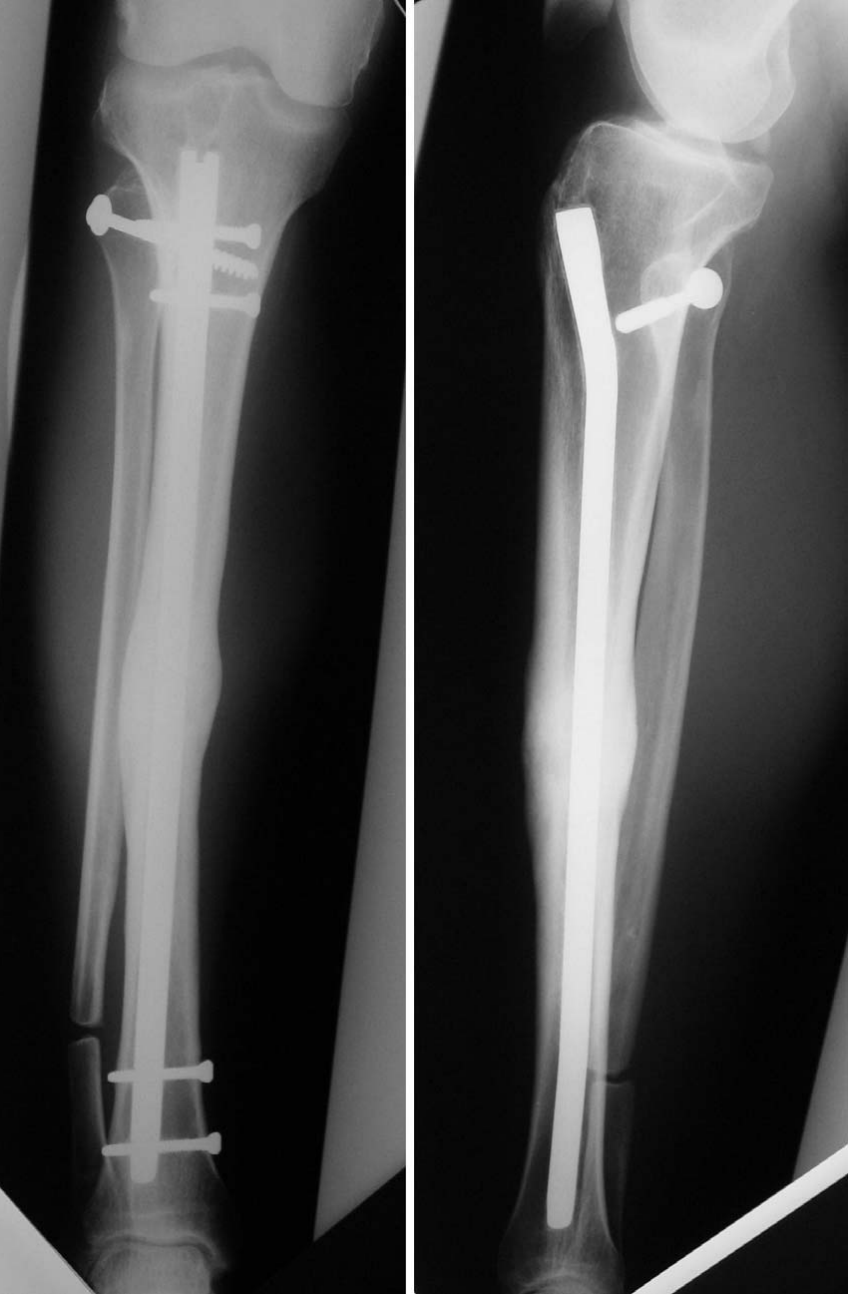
Anteroposterior and Lateral X-ray. The open reduction and internal fixation of the tibiofibular dislocation and the intramedullar nail as the treatment of the tibial shaft fracture. The distal fibular osteotomy is done. The consolidation of the fracture is achieved.
